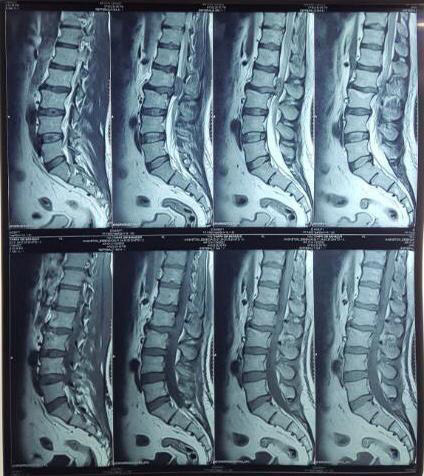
T2 sagittal section MRI of Lumbo Sacral Spine.
